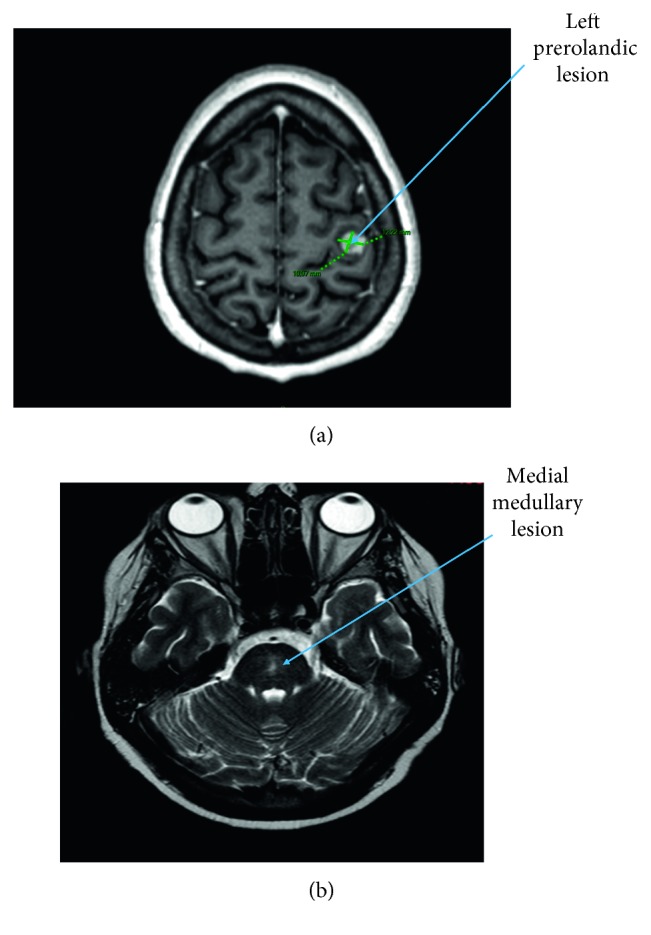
Brain MRI showing left prerolandic lesion and medial medullary hypersignal. (a) Prerolandic lesion with homogeneous corticosubcortical ring enhancement. (b) Medial medullary hypersignal in T2 and flair.
